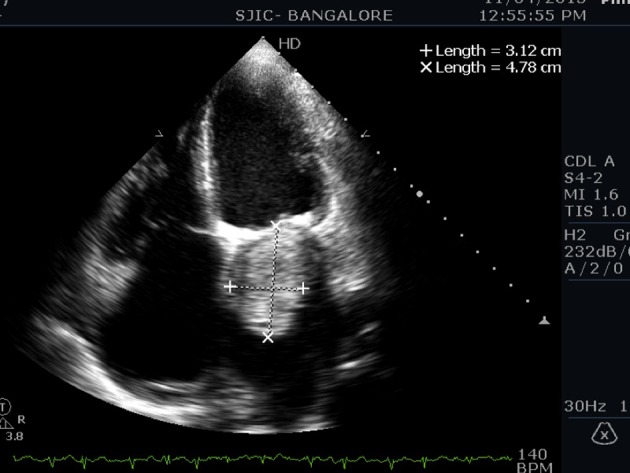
Apical four-chamber view showing a 4.7 × 3.2 cm myxoma.
